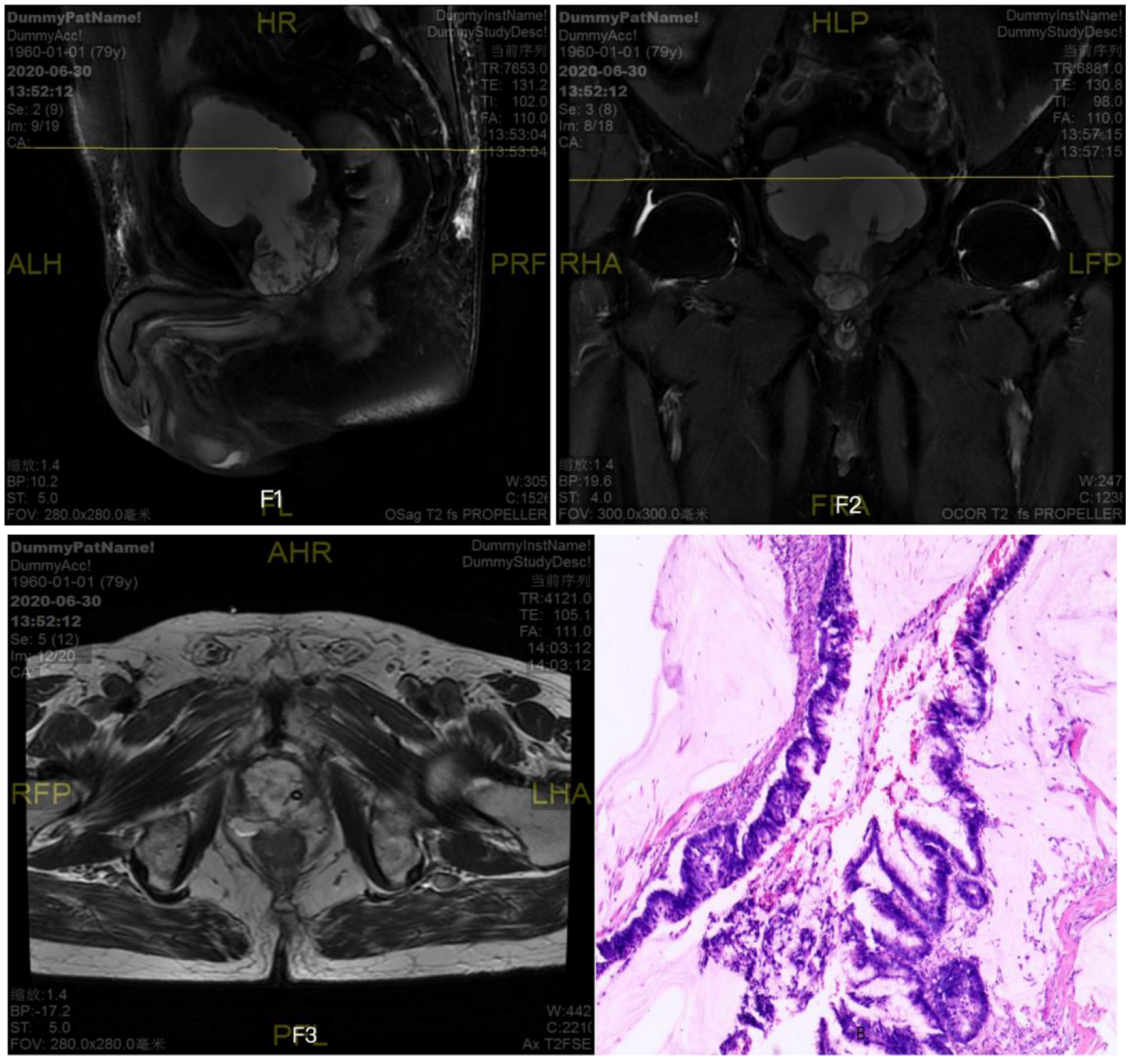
Prostate MRI (scanning)+prostate adenocarcinoma with mucinous carcinoma differentiation (cancer cells arranged in adenoid pattern and rich in mucus).
